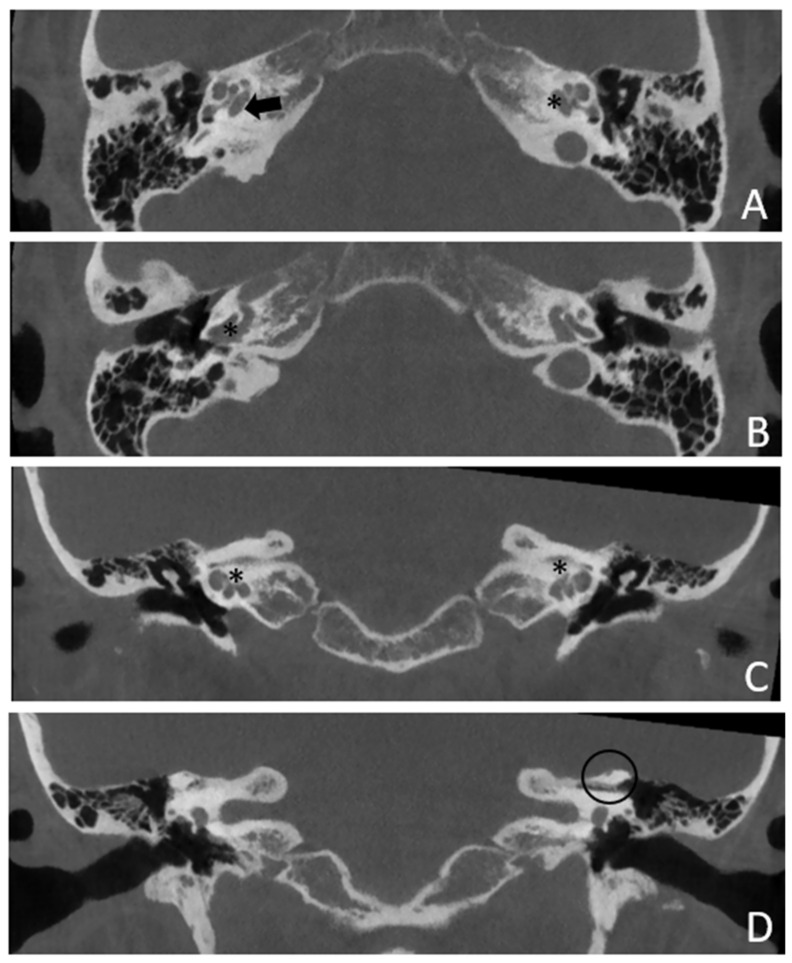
CT images of patient #3 with bilateral otosclerosis and SSCD of the left ear. (A,B), axial plane; (C,D), coronal plane. A cavitating osteorarefation around the cochlea is clearly visible. The communication of the cavity with the cochlea is indicated by asterisks. The arrow indicates the origin of the cavity from the IAC. The circle indicates the dehiscence of the SSC on the left side.
